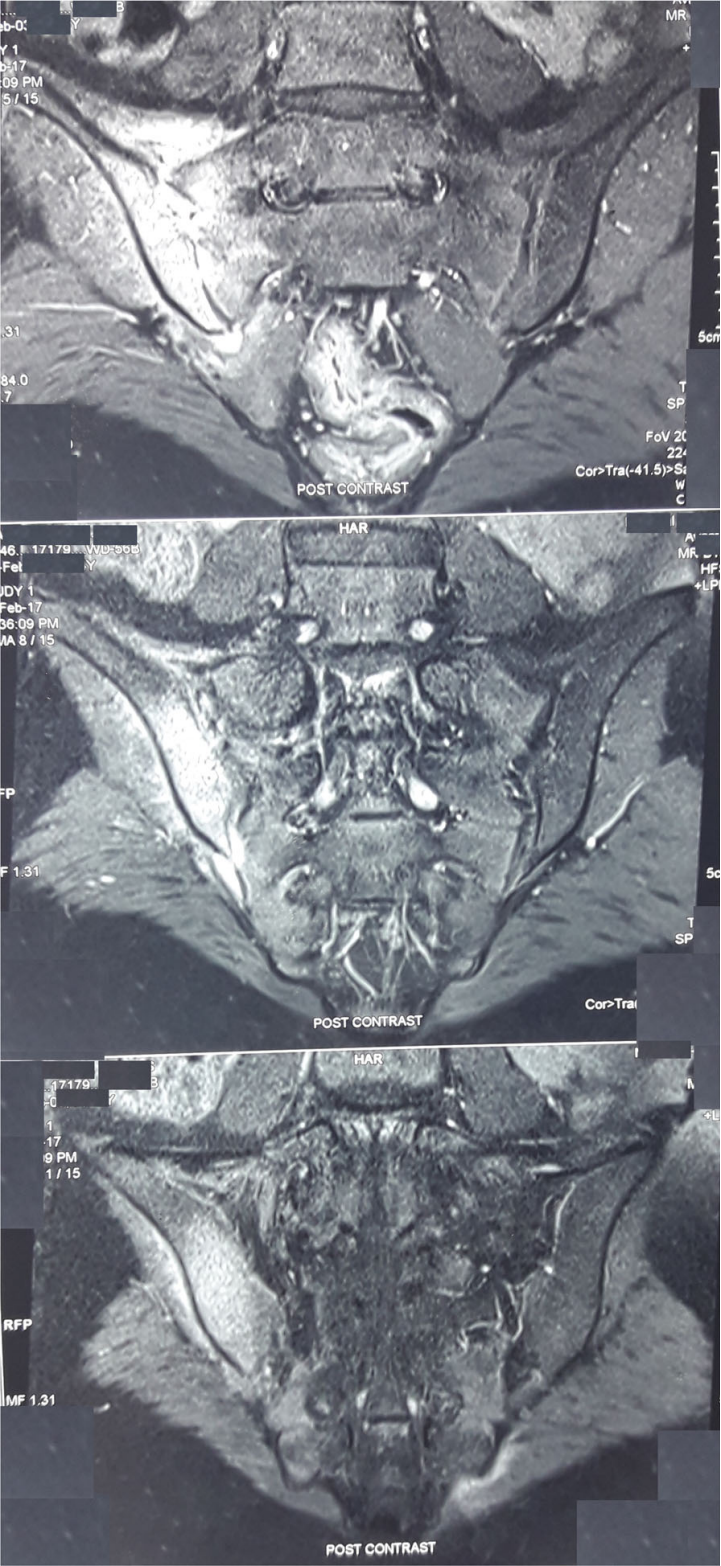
Magnetic resonance imaging of the sacroiliac joint showing high T2 signal intensity around right sacroiliac joint with surrounding marrow edema and reactive bony changes suggestive of acute right-sided sacroiliitis
